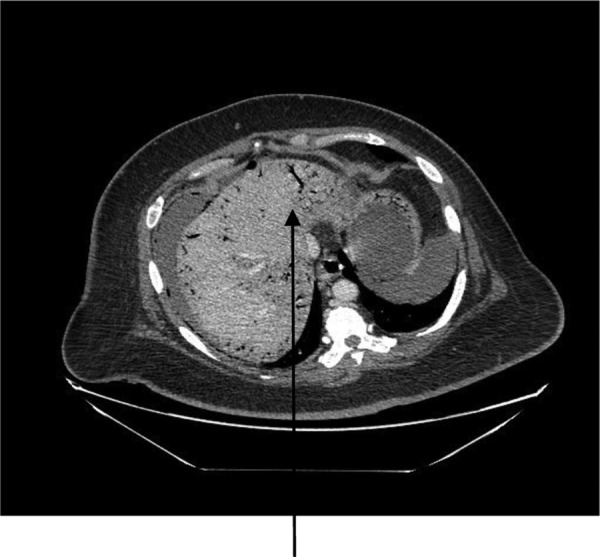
CT abdomen showing diffuse portal venous gas in the liver.
